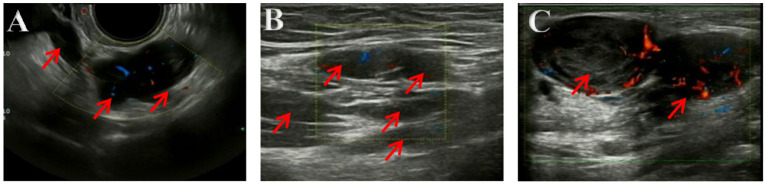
(A–C) Ultrasonography imaging. Multiple solid masses in the subcutaneously muscular layer, with size of 2.8 × 2.8 × 1.8 cm in bigger mass. Color Doppler flow imaging (CDFI) showed a strip-like blood flow signal.
